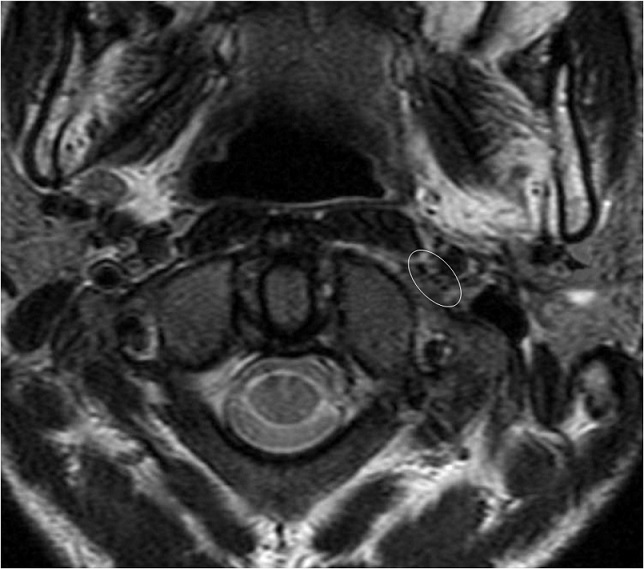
The upper cervical MRI on T2 weighted image demonstrate the fourth lower cranial nerves (circle) which appear as black points with high signal intensity around, related to perinerve oedema. On the other side the CN are not visible
